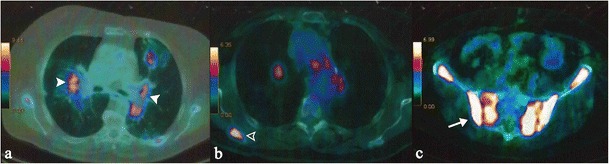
Skeletal sarcoidosis in a 71-year-old woman. Bilateral thoracic hilar lymphadenopathy is shown on PET-CT (Fig. 19a, *white arrowheads*). Increased sites of metabolic activity are also recognizable in the right scapula (Fig. 19b), in the sacrum and in the iliac crest (Fig. 19d). In presence of thoracic features of sarcoidosis, bone sites of increased uptake can be interpreted as “skeletal sarcoidosis”
